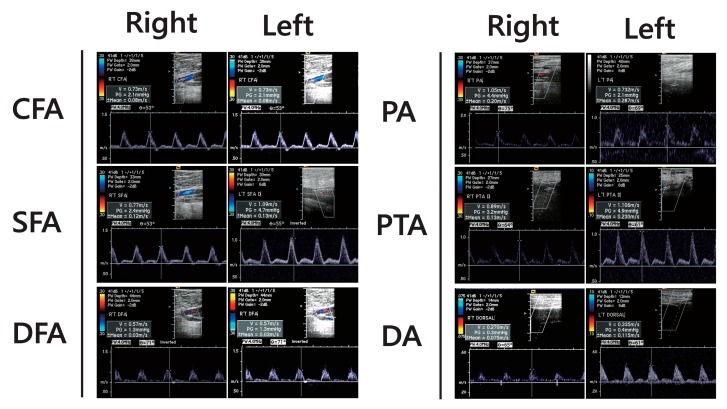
The doppler ultrasound of extremities revealed no significant decrease of blood flow or abdominal atherosclerosis in vessels. Right: right leg; Left: left leg; CFA: common femoral artery; SFA: superficial femoral artery; DFA: deep femoral artery; PA: popliteal artery; PTA: posterior tibial artery; DA: dorsalis pedis artery.
